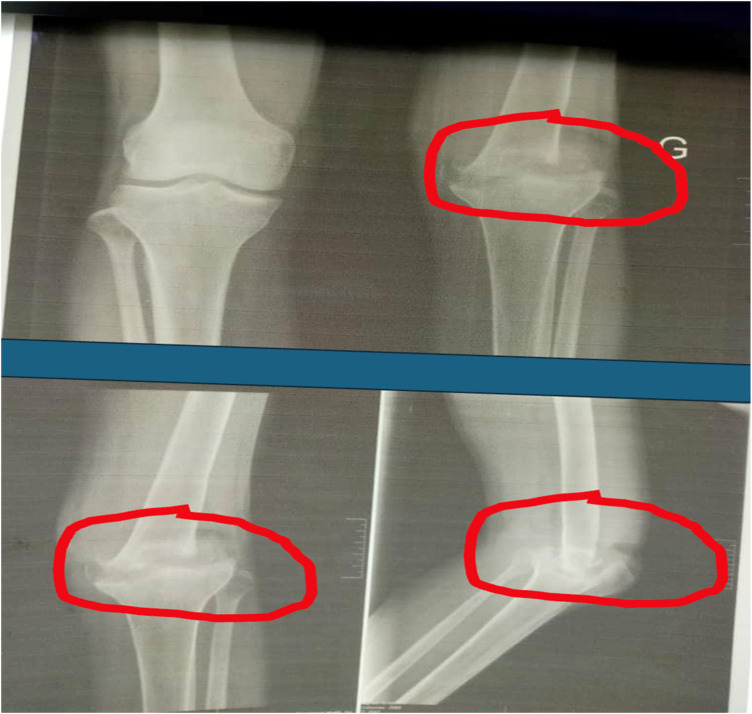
Anteroposterior and lateral radiographs of the left knee in a 17-year-old male, three months after untreated sports-related trauma. Red circles highlight areas of significant regional demineralization (disuse osteopenia) involving the distal femur and proximal tibia, with cortical irregularities at the distal femoral metaphysis consistent with a healing traumatic lesion. Note the diffuse reduction in bone density in the periarticular regions compared to adjacent diaphyseal segments, reflecting three months of functional underloading. “G” marker indicates left side (Gauche). No significant articular displacement or physeal arrest is observed.
